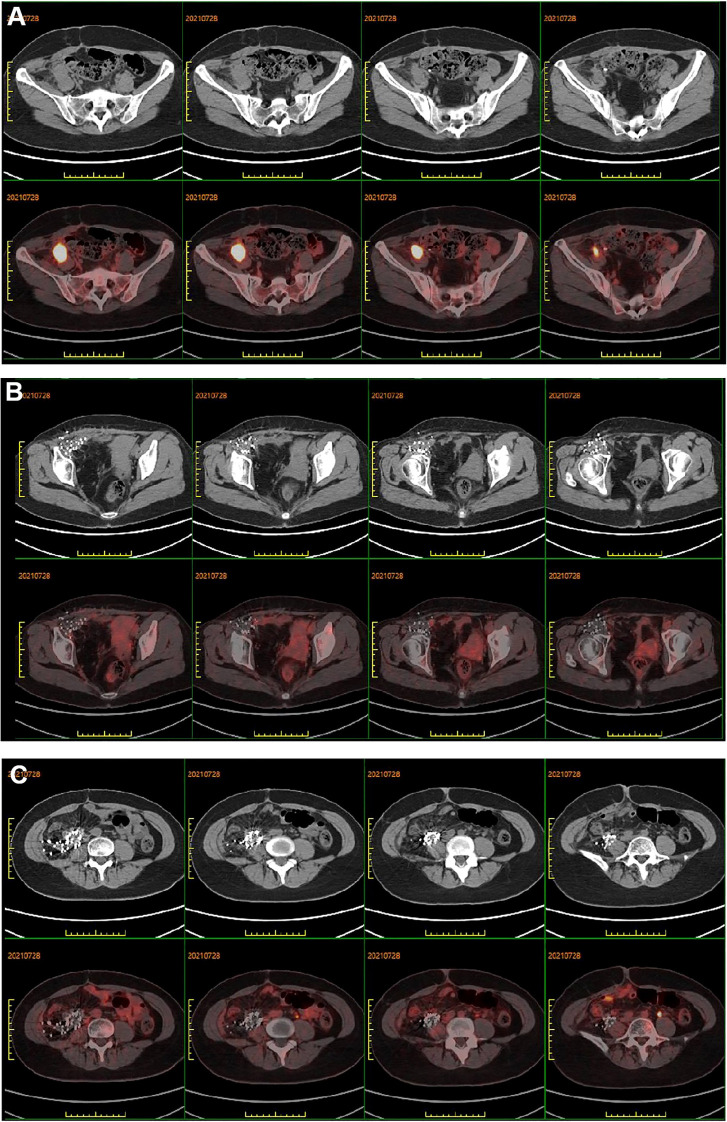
(A) PET-CT showed peripheral elevated FDG avidity in the right pelvic cavity. (B, C) PET-CT scan demonstrated no peripheral elevated FDG avidity in the right groin and right abdomen after 3 years of iodine-125 seed implantation, respectively.
